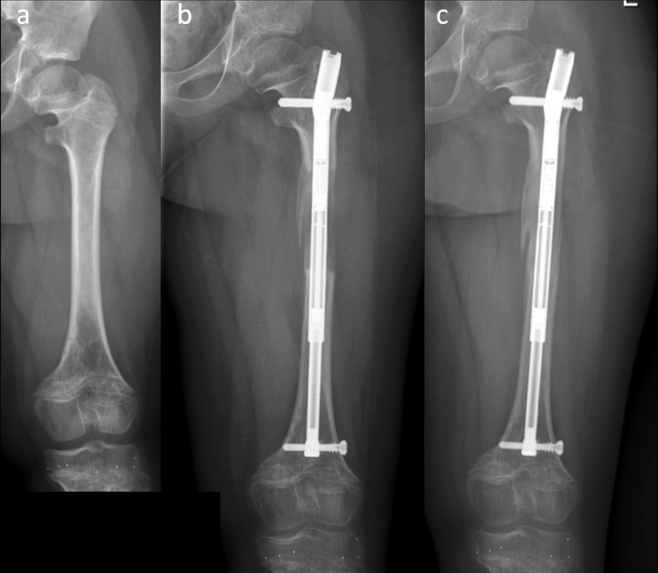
A 12-year-old girl with achondroplasia. She underwent consecutive 50 mm lengthenings of both femurs with the shortest available Precice nail (16.5 cm). Radiographs preoperatively (a), when lengthening was completed (b) and after consolidation (c). The nail has only one locking option proximally and distally to allow for 50 mm lengthening, despite the shortness of the nail. The patient’s femurs had not been lengthened earlier. Lengthening indices for both femurs were 0.6 months/cm, the fastest healing of all procedures included in the current study.
